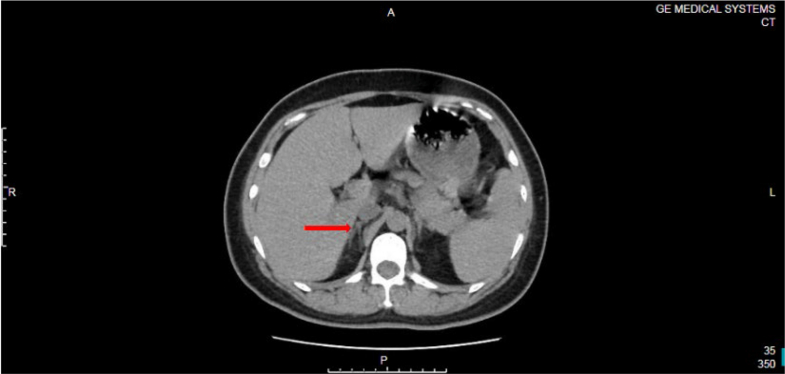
Noncontrast Chest Computed Tomography Scan From October 29, 2021, Incidentally Capturing the Adrenal Region (Arrows), Showing no Definite Nodule
